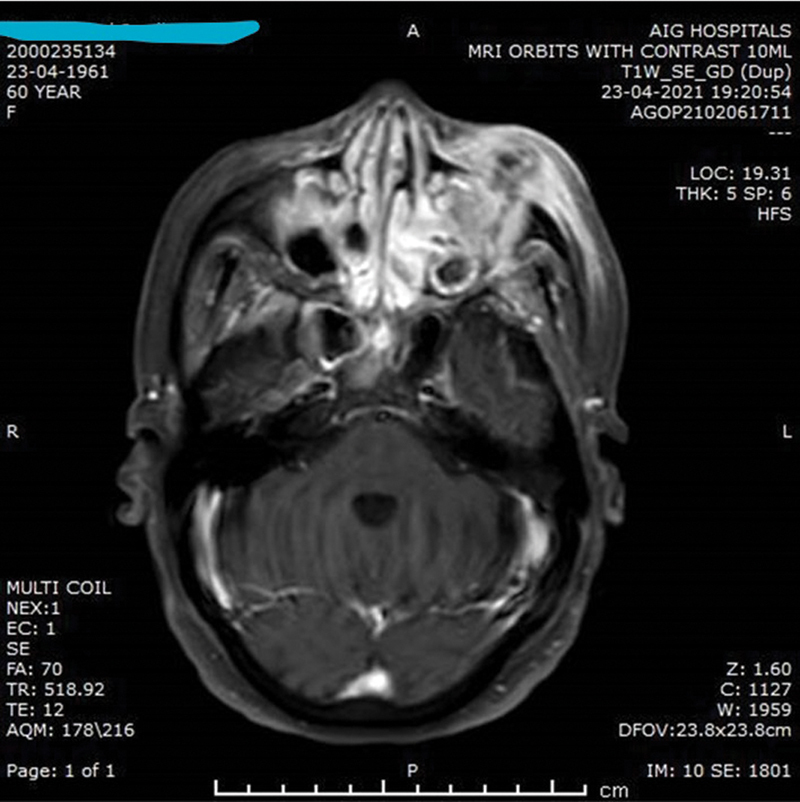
MRI OF PNS AND ORBITS Suspected face of Mucormycosis involving PNS and Left Orbit. Heterogenously enhancing contents in left maxillary sinus with extension into left orbit and soft tissues on left side of face. Similar heterogenously enhancing contents in bilateral ethmoidal air cells,bilateral sphenoid sinuses (R>L) and right maxillary sinus.
